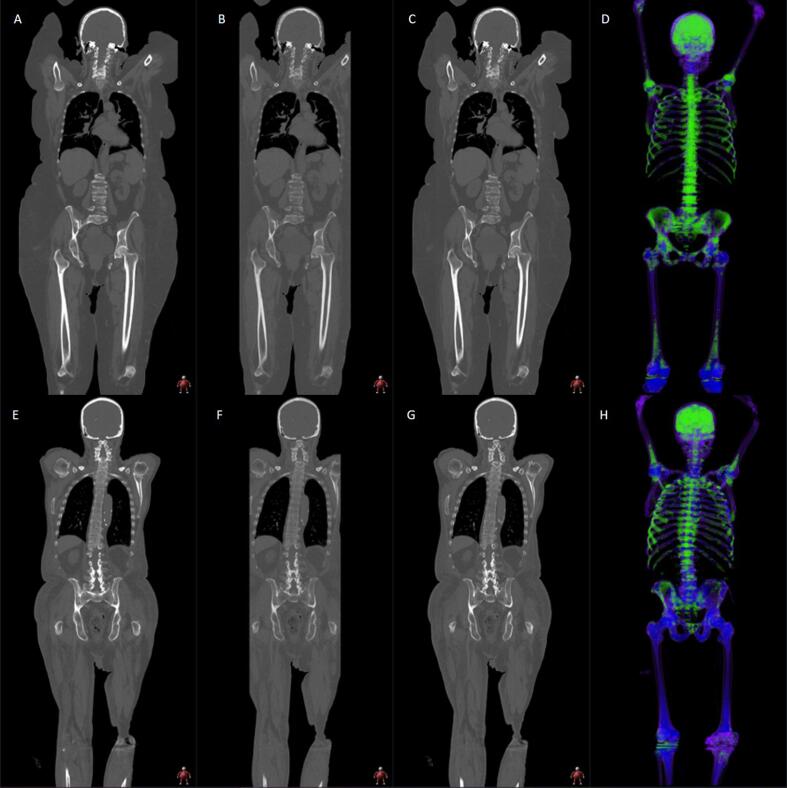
60-year old female with a new diagnosis of myeloma (top row). Representative dual-energy CT images show multifocal disease and relatively diffuse marrow infiltration, particularly within the axial skeleton. Reconstructed coronal 90kVp (A); 150 kVp (B); weighted-average 120 kVp images (C); and colour-coded whole body rendered image (green representing infiltration) (D). 88-year old male with a new diagnosis of myeloma (bottom row). Representative dual-energy CT images show multifocal disease but patchy heterogeneous marrow infiltration. Reconstructed coronal 90kVp (E); 150 kVp (F); weighted-average 120 kVp images (G); and colour-coded whole body rendered image (green representing infiltration) (H).
